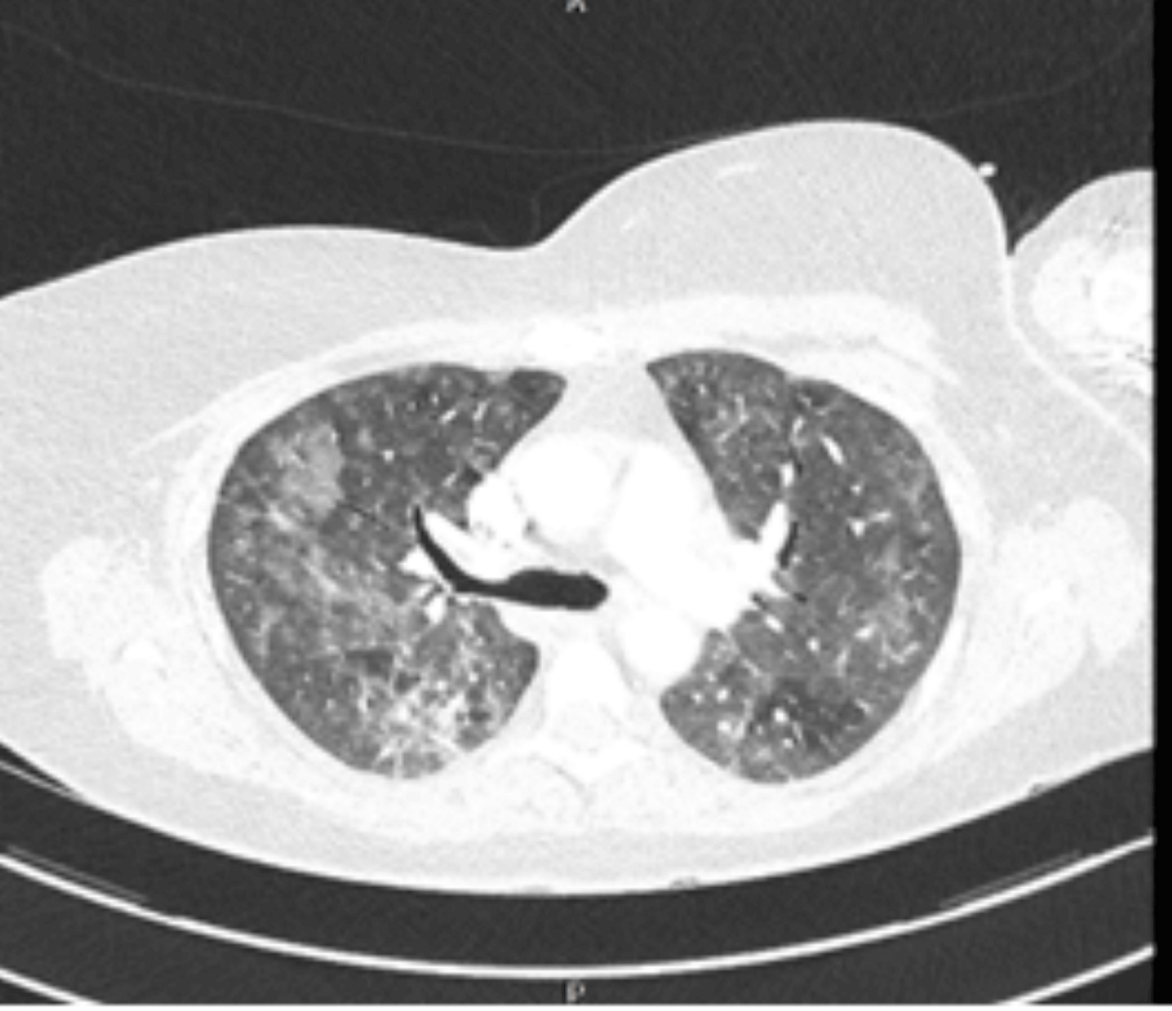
Computed tomography scan of chest revealing bilateral infiltrates
